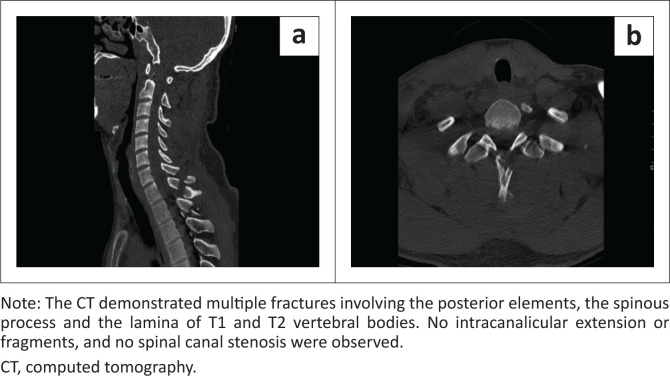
CT C-spine (a) sagittal reconstruction and (b) axial view of a patient who presented with neurological fall out, quadriplegia.
